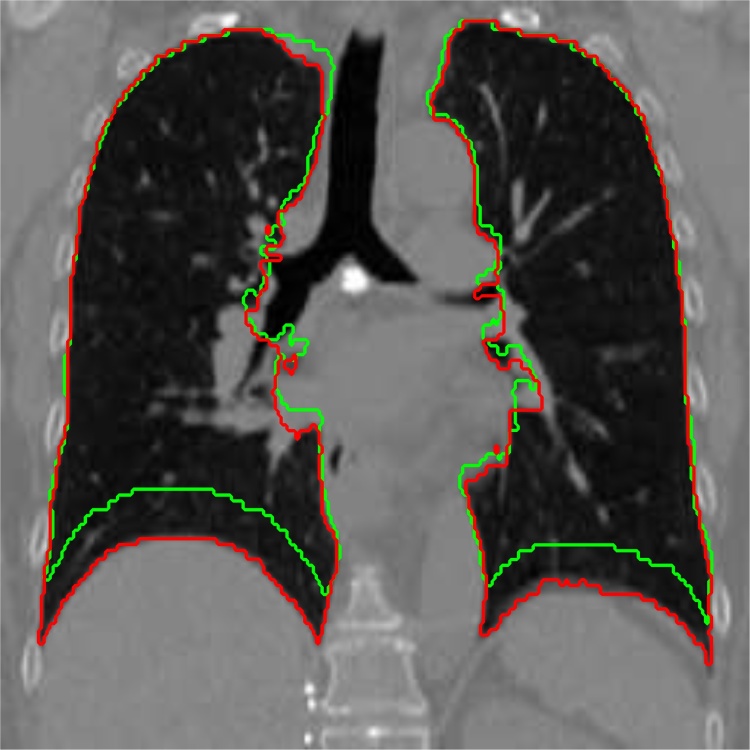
Example of superimposed lung segmentations in inhale state (red) and exhale state (green). Segmentation was performed by experts. The image comes from publicly available *Dir-Lab* set of CT data described in Castillo et al. (2009). Function *ϕ*_*L*_ introduced in Eq. (7) takes value 1 inside the red contour and value 0 outside of it. (For interpretation of the references to color in this figure legend, the reader is referred to the web version of this article.)
